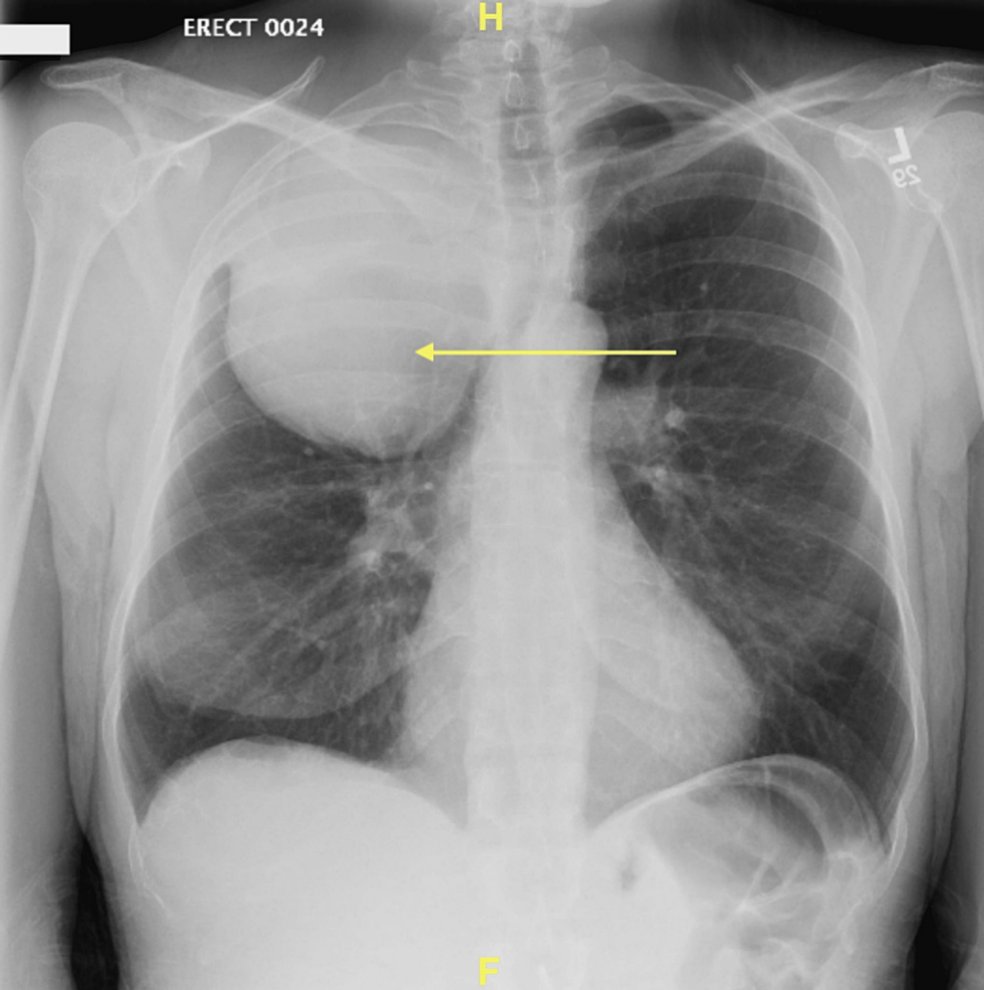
Chest X-ray demonstrating a circumscribed mass in the right upper thorax projecting over the right upper lobe.
